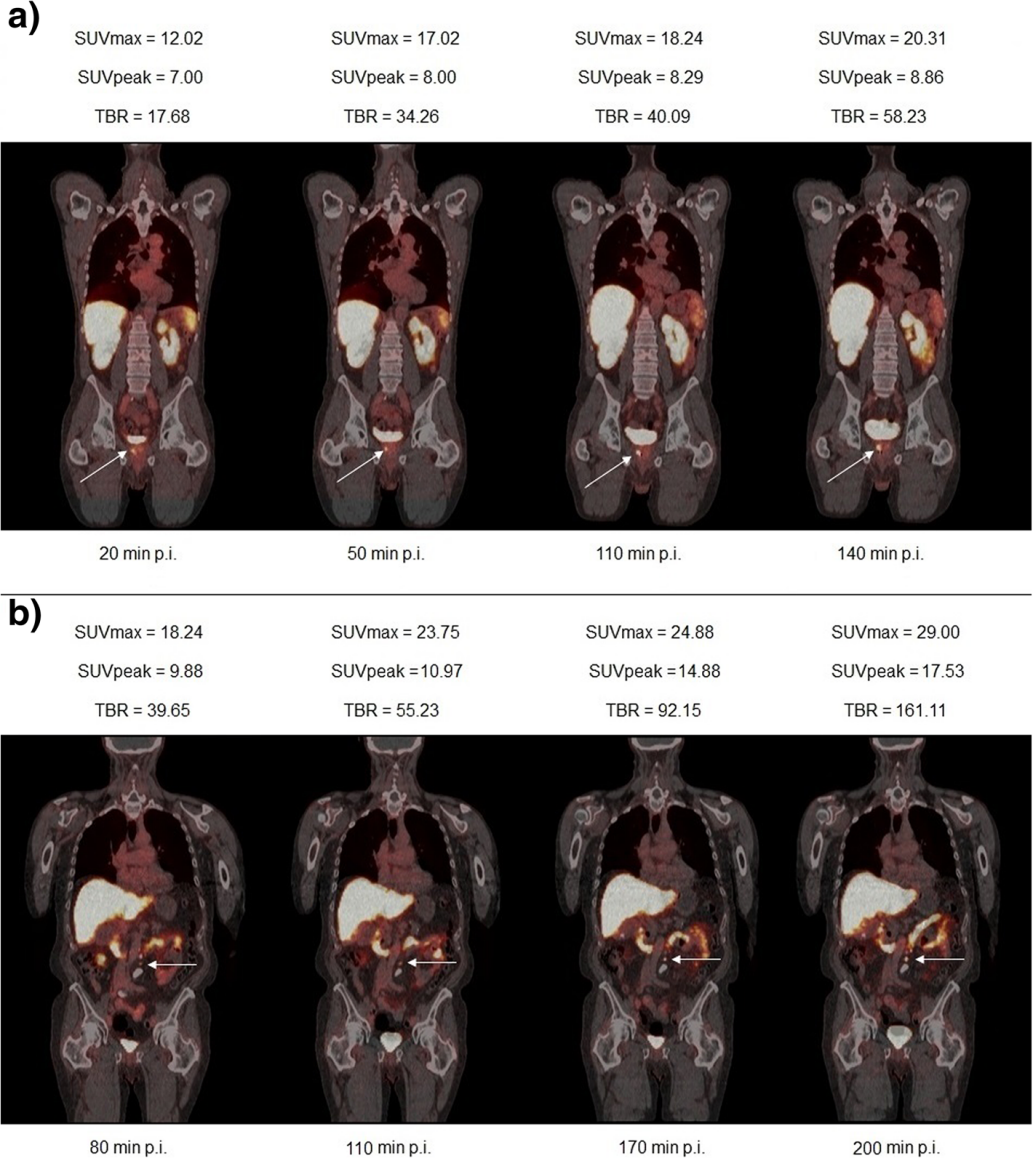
a [^18^F]-JK-PSMA-7 PET/CT scans obtained at 20 min, 50 min, 110 min, and 140 min p.i. and corresponding values for the SUV_max_, SUV_peak_, and TBR of one suspicious lesion within the prostate fossa (arrow). b [^18^F]-JK-PSMA-7 PET/CT scans obtained at 80 min, 110 min, 170 min, and 200 min p.i. and corresponding values for the SUV_max_, SUV_peak_, and TBR of a suspicious retroperitoneal lymph node (arrow). Here, we have quantified the middle lymph node from a PSMA-positive lymph node chain
